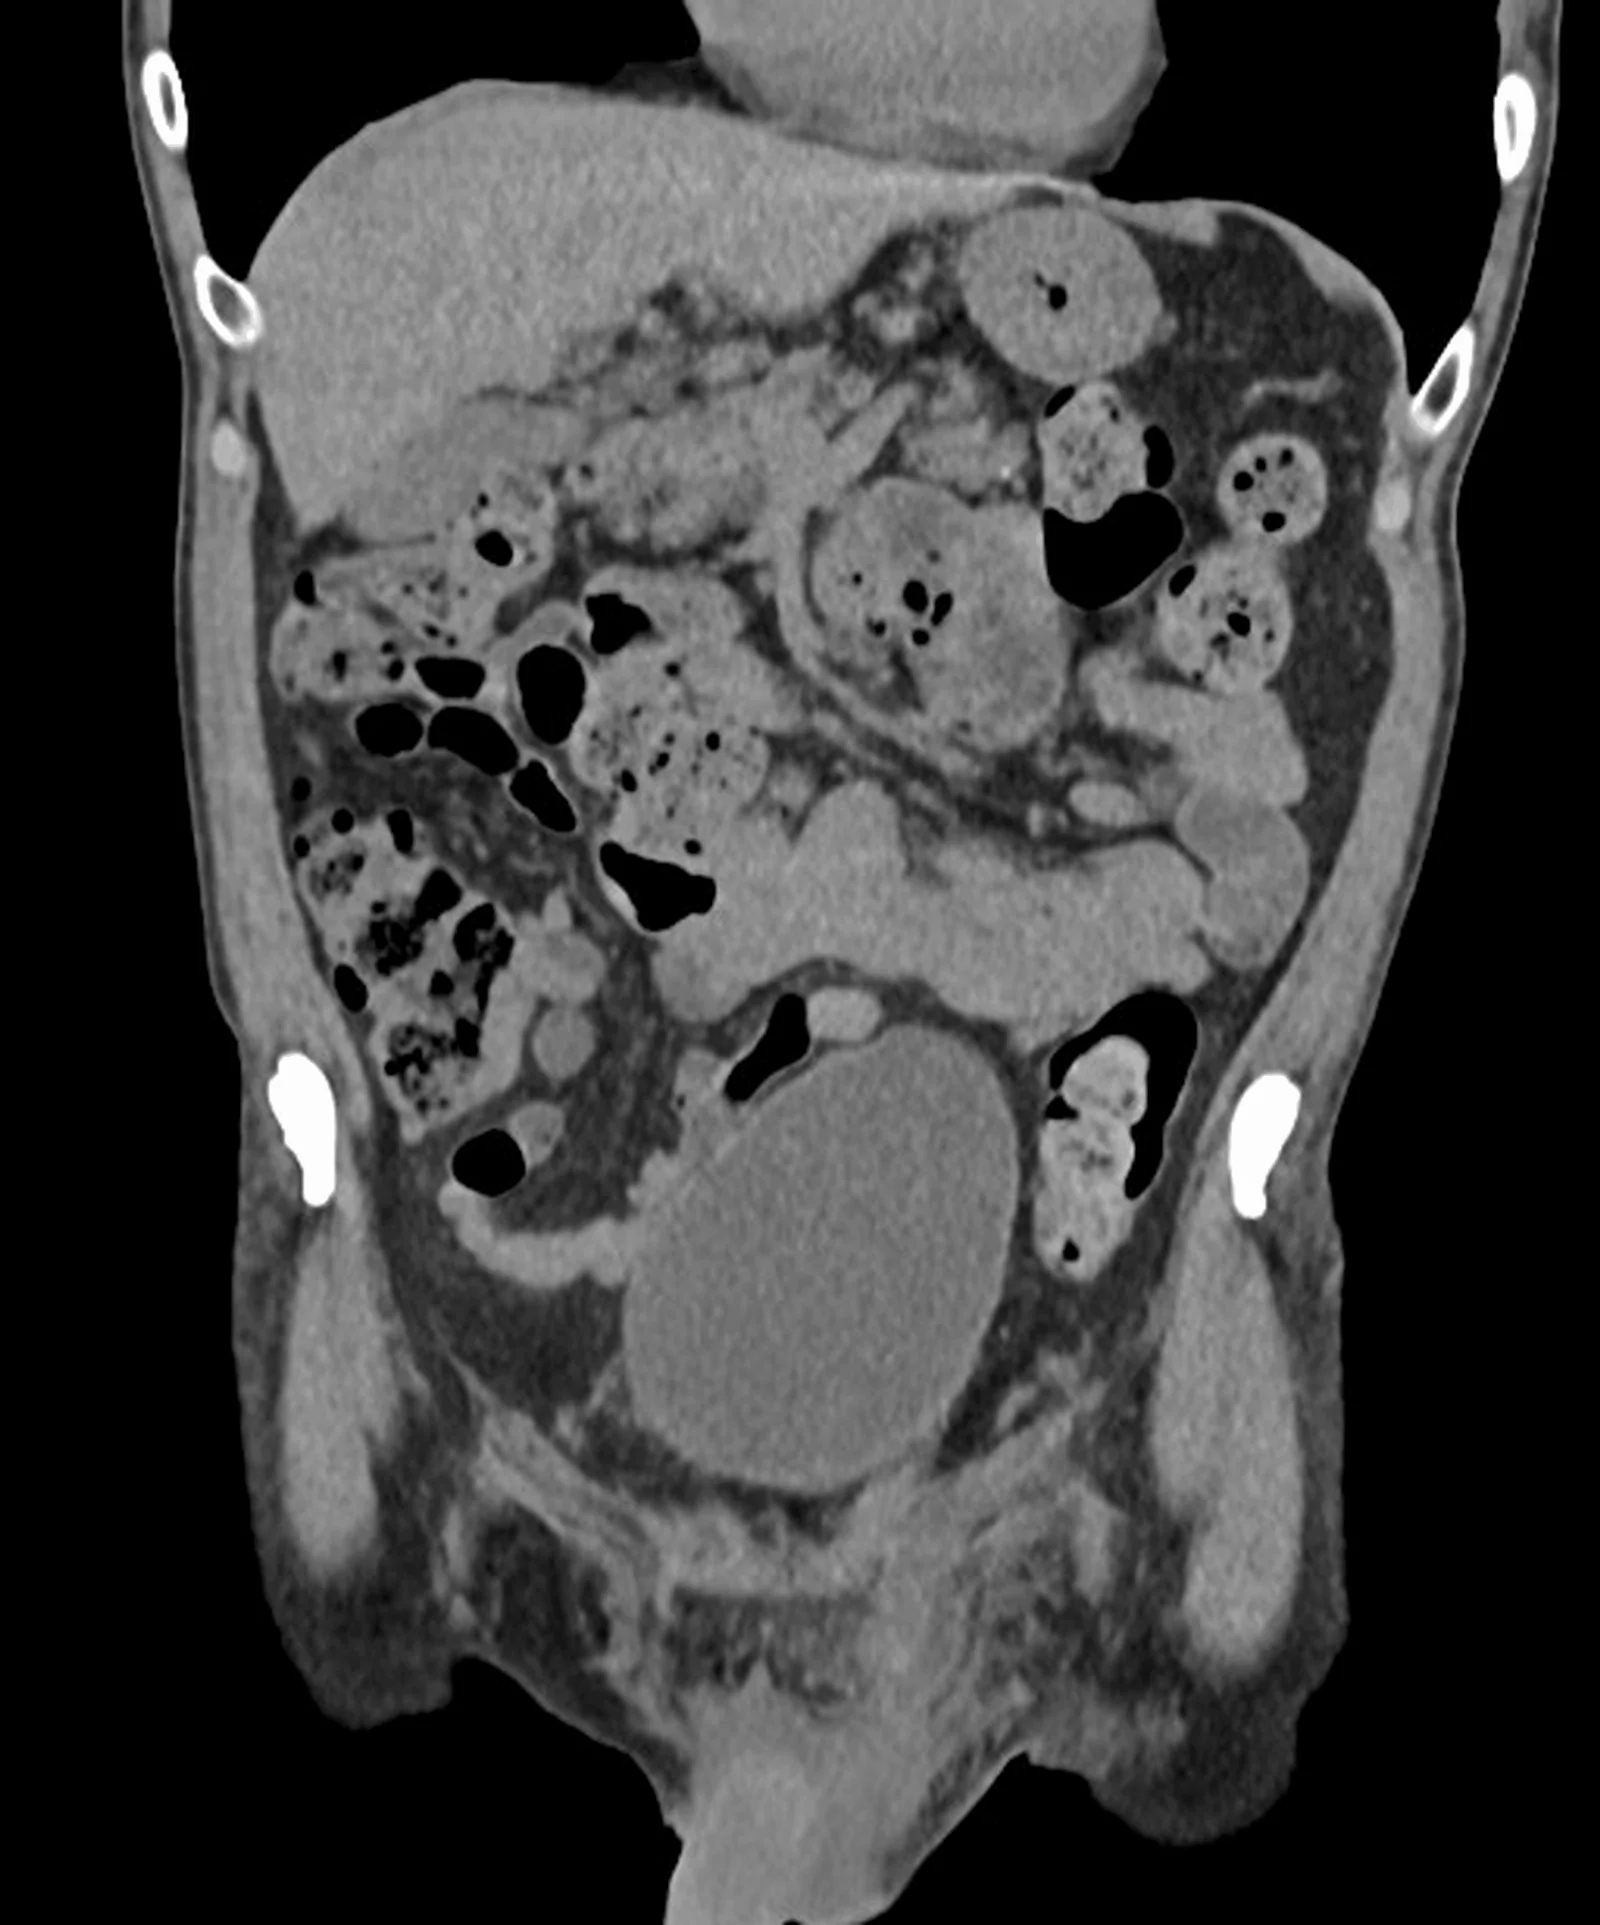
Coronal non-contrast computed tomography of the left inguinal region Coronal non-contrast computed tomography (CT) reconstruction demonstrating a 27 × 28 × 53 mm fat-containing soft-tissue lesion within the left inguinal canal, initially interpreted as an incarcerated recurrent inguinal hernia. Associated ipsilateral ureterohydronephrosis secondary to distal ureteral stenosis is also demonstrated. Surgical exploration subsequently identified the lesion as an abscess adjacent to the vas deferens rather than a recurrent inguinal hernia.
